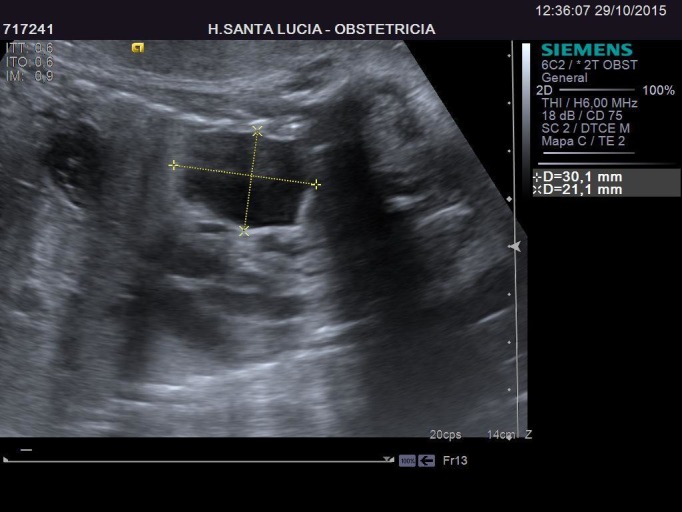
Ultrasound - Sagittal View This figure shows the size of the congenital adenomatoid malformation. Notice that the stomach is in the abdomen, below the thorax.
